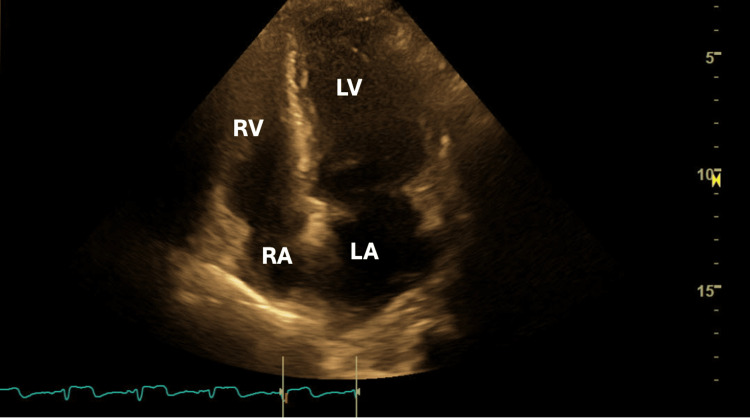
An apical four-chamber view showing the left ventricle (LV), left atrium (LA), right ventricle (RV), and right atrium (RA)
